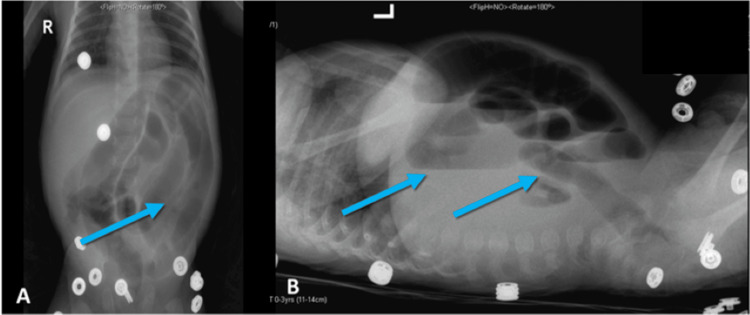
X-ray abdomen, two views: A. The anterior-posterior view showed dilated bowel loops and some pneumatosis intestinalis (blue arrow). B. The lateral decubitus view showed dilated bowel loops and some pneumatosis intestinalis (blue arrows)
